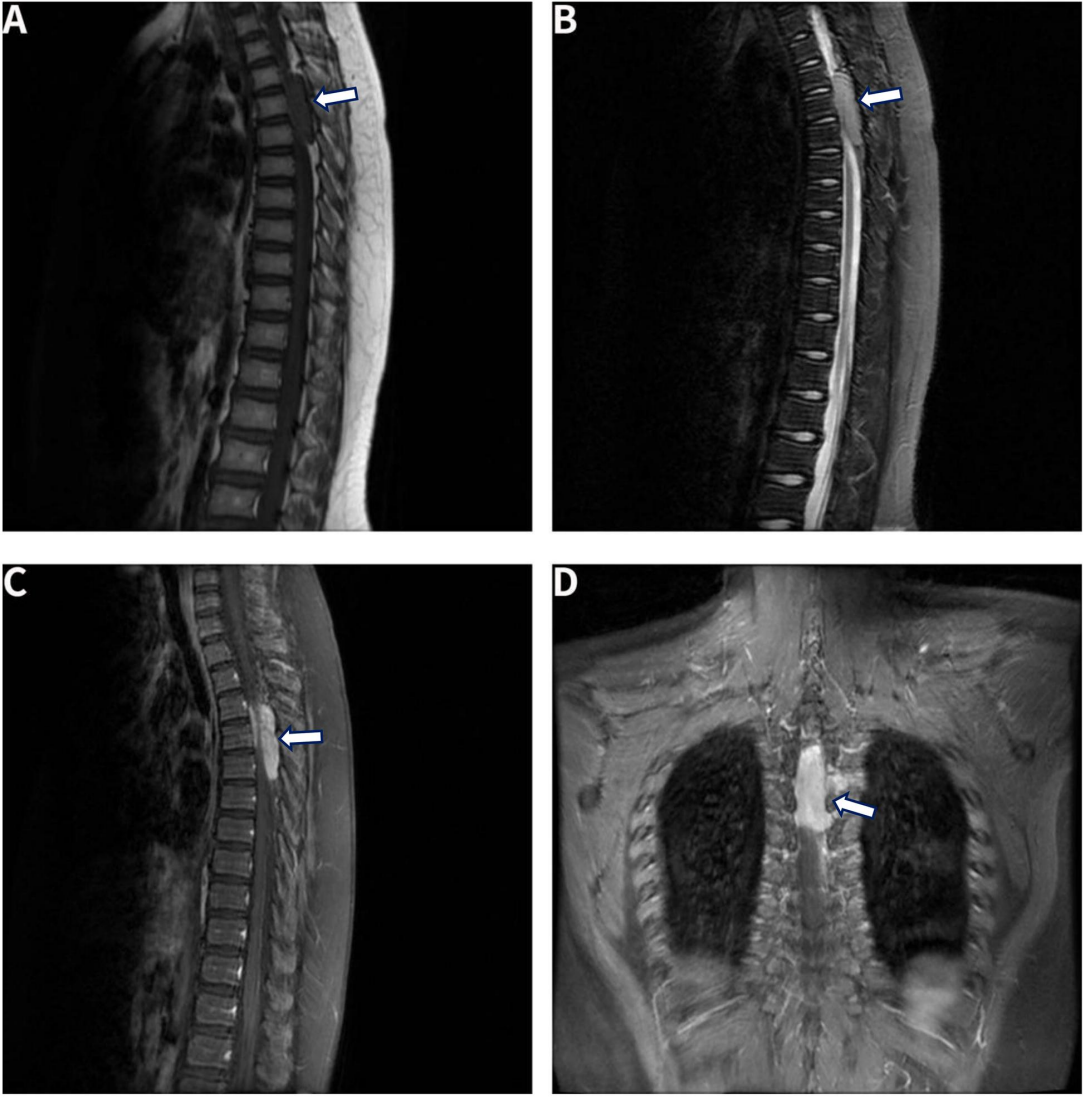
MRI scan of the thoracic spine showed an oval-shaped, long T1 and long T2 signal shadow within the extradural space at the T3–5 level. (A) T1-weighted image. (B) T2-weighted image. (C) and (D) Sagittal and coronal enhanced MRI images. Arrows indicate the locations of the lesions.
